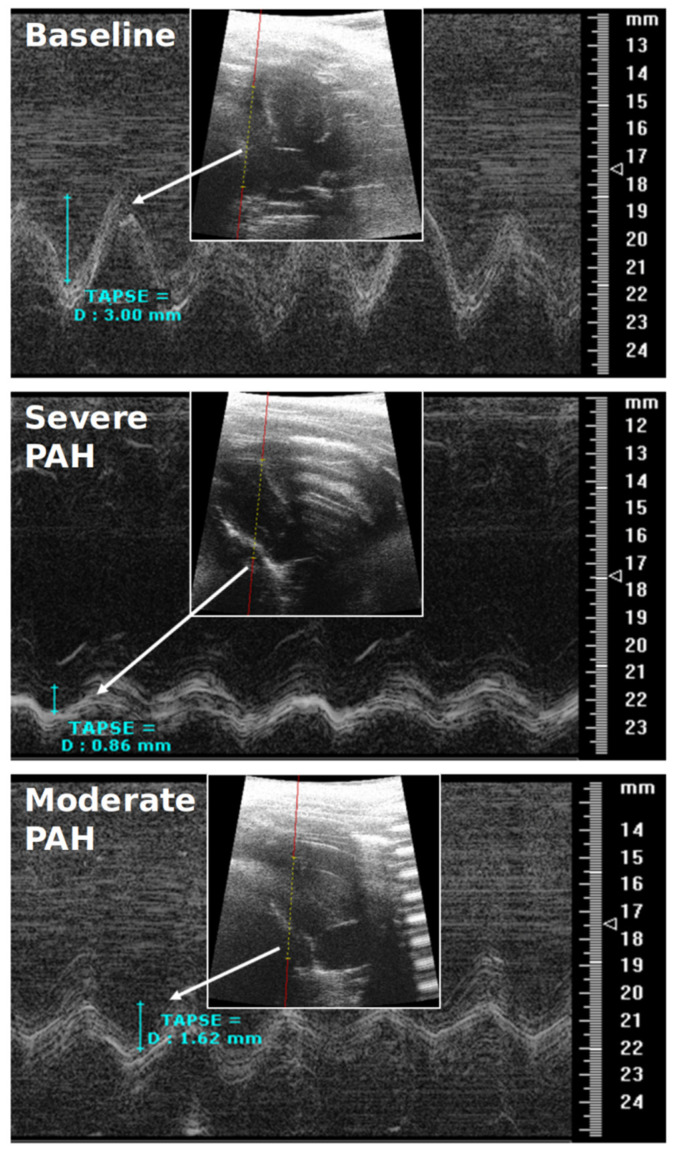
Representative transthoracic echocardiography (TTE) images showing the tricuspid annular plane systolic excursion (TAPSE) in baseline, moderate, and severe pulmonary arterial hypertension.
